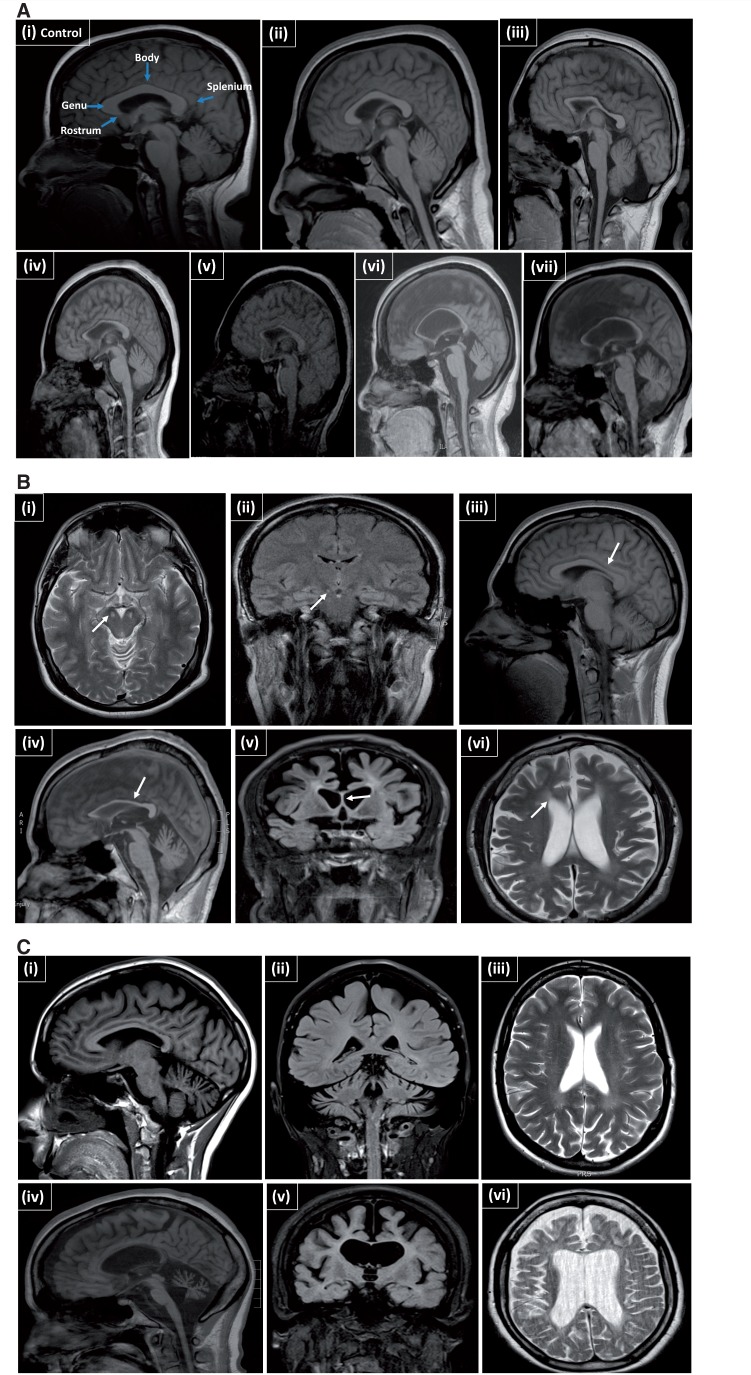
MRI features in patients with complex HSP.
